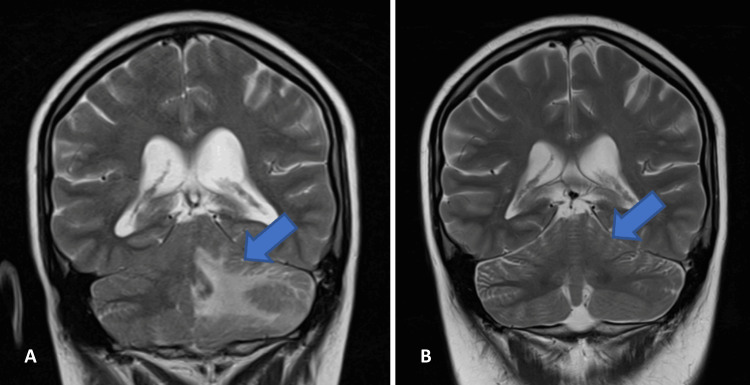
Comparative images of the brain MRI at admission (A) and one month after treatment initiation (B). The blue arrow indicates the infiltrative brain lesion. Abbreviation: MRI, magnetic resonance imaging.
